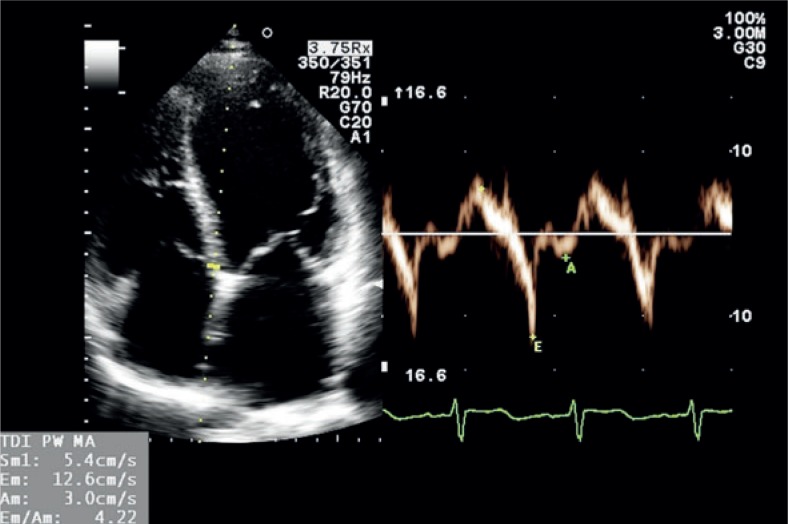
Measurement of tissue Doppler velocities on the mitral annulus from the apical four chamber view showing depressed left ventricular systolic function. (x = systolic velocity, × E = early diastolic velocity, × A = atrial contraction velocity).
